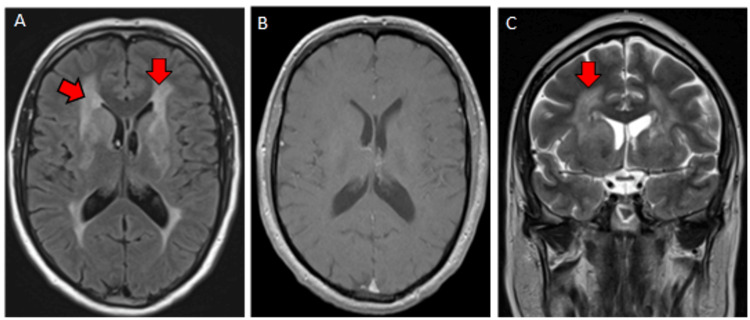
MRI of the brain showing leukoencephalopathy Magnetic resonance imaging (MRI) of the brain shows a pattern of diffuse but asymmetric T2/fluid-attenuated inversion recovery (FLAIR) (panels A and C) deep white matter hyperintensity involving the basal ganglia, including the caudate nuclei, without contrast enhancement on the T1 contrasted sequence (panel B).
